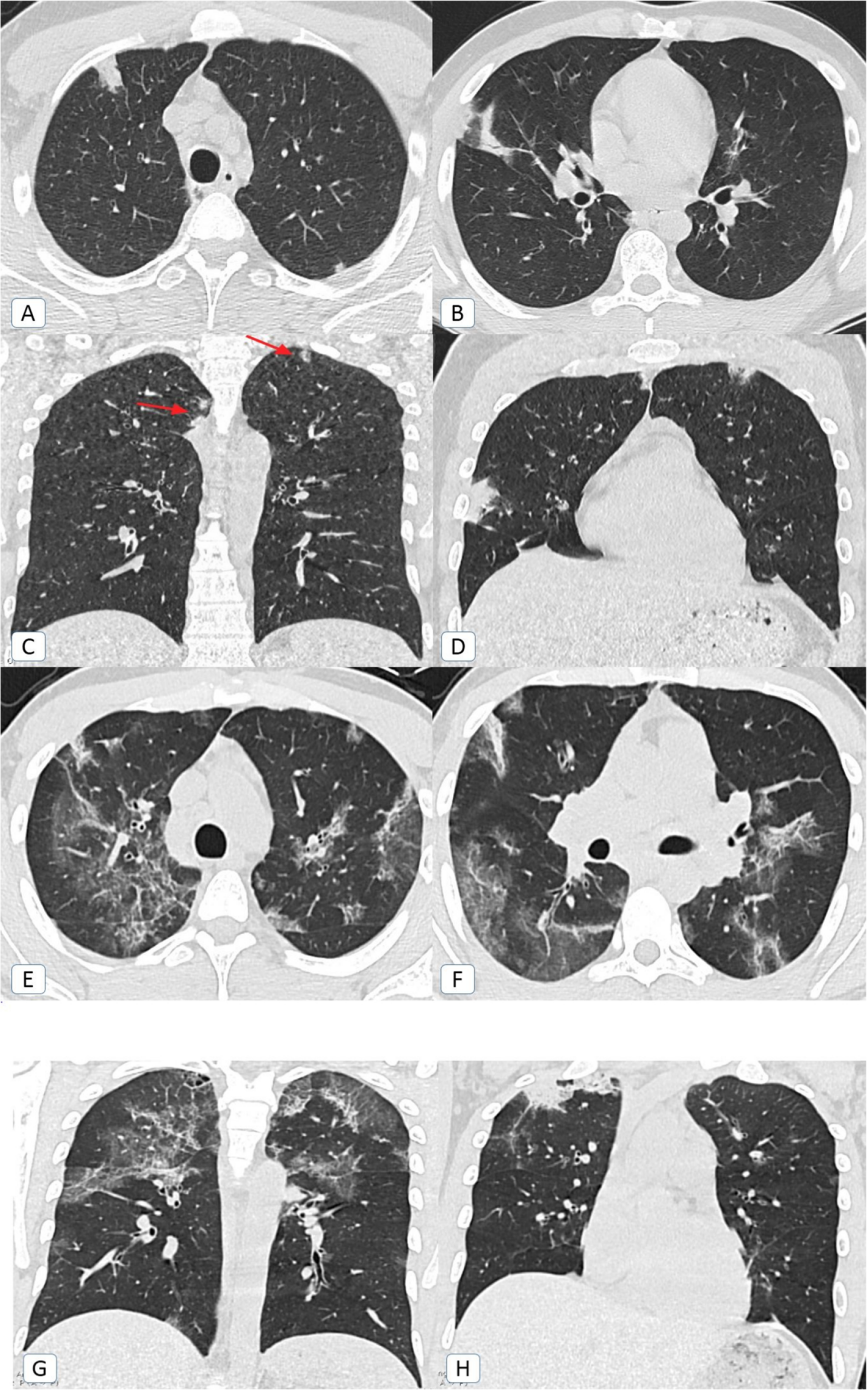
COVID-19 reinfection: A 35-year-old male COVID-19 patient. A, B Axial, and C, D coronal chest CT (lung window) during the mild first infective episode at the beginning of October 2020, showing small bilateral upper lobar mixed ground glass and consolidative patches. E, F Axial and G, H and coronal chest CT (lung window) during the more severe reinfection at the end of March 2021, showing widespread bilateral upper and lower lobar mixed ground glass and consolidative patches with fibro-atelectatic changes. Also noted right apical mild cystic changes as a sequel to the previous infection
